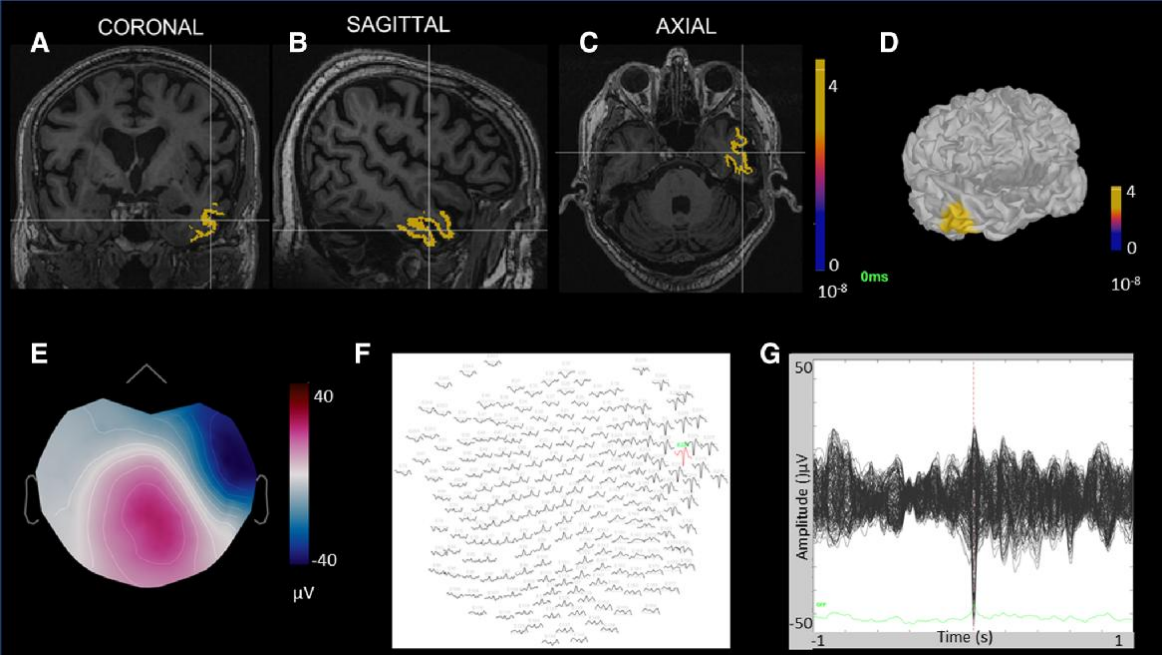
Electronic source imaging in one patient with aMCI. The enclosed area marked with the perpendicular lines identifies the sources at the time peak of one epileptiform discharge, shown on individual MRI viewer on (A) coronal, (B) sagittal and (C) axial view. (D) Cortical sources’ activation at spike’s time peak on 3D model, Standardized low resolution brain electromagnetic tomography (sLORETA) method. (E) Voltage topography map that shows the dipole at the spike’s peak. (F) Monopolar HD-EEG layout, in red the channel with the max amplitude at the spike’s peak. (G) Butterfly plot of the spike, −1000 to +1000 ms.
